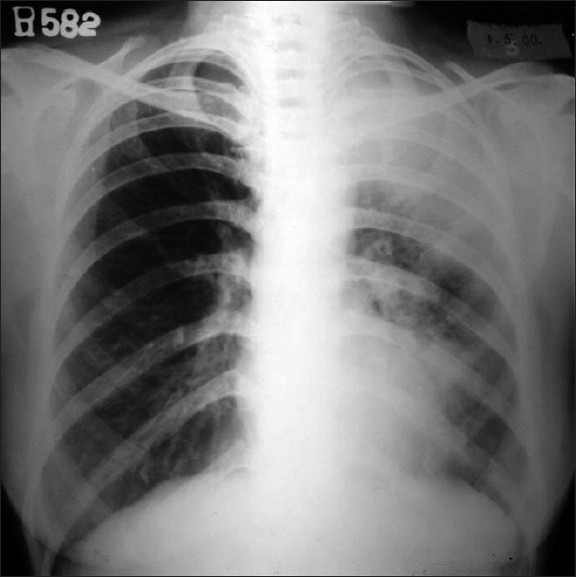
Chest X-ray of a 56-years-old lady with an acute exacerbation of asthma showing left upper lobe collapse-consolidation along with left paracardiac opacity
